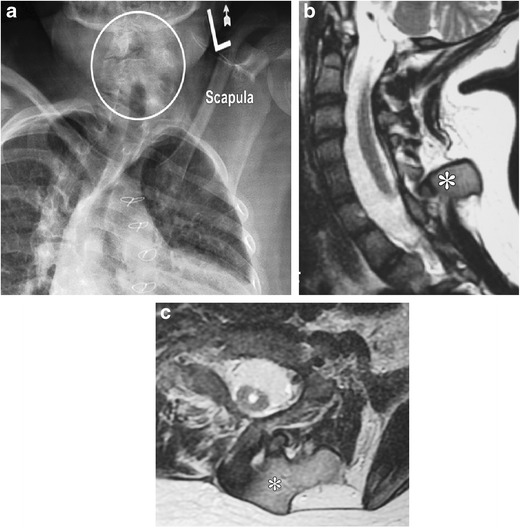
a-c: Klippel-Feil abnormality with Sprengel shoulder: Frontal radiograph of the neck and upper chest in an 11 year old demonstrates marked elevation of the left scapula. Also seen are segmentation anomalies of the cervical spine (demarcated by *circle* on *a*). Sagittal (*b*) and axial (*c*) T2-weighted MR images demonstrate a large bony omovertebral bar (marked by *asterisks* on both images) extending between the medial border of elevated scapula and spinous process of a lower cervical vertebra
